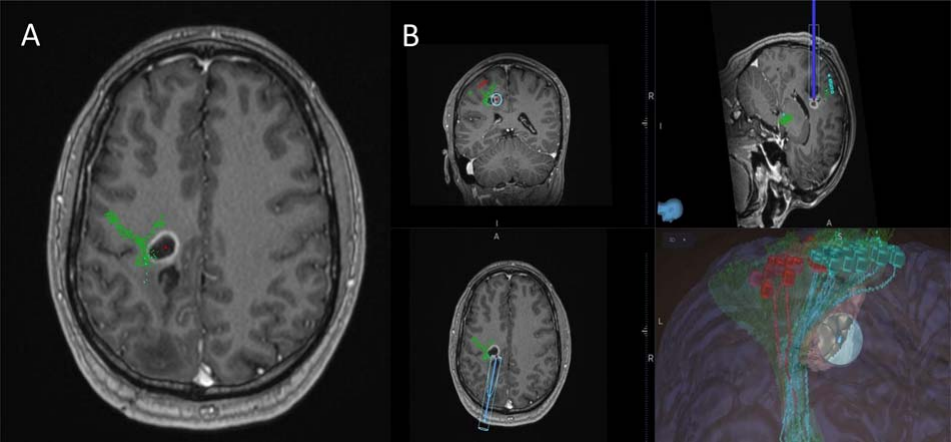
(A) Axial T1-weighted image with gadolinium showing the lesion with imposed DTI tractography of the CST; (B) planned trajectory for insertion of the tubular retractor guided by the preoperative integrated anatomical and functional motor mapping.
